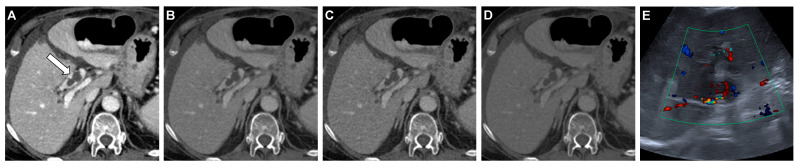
Axial DECT images of a 90-year-old woman with liver cirrhosis and portal vein thrombosis (*arrow*). Noise-optimized virtual monoenergetic imaging (VMI+) series at 40 keV (A) show higher vascular attenuation of the portal vein and a better delineation of the thrombus compared to 60 keV VMI+ (B), 60 keV VMI (C), and standard linearly blended M_0.6 images (D). On color duplex ultrasonography, some color signals are visible within the boundaries of the thrombus (E).
